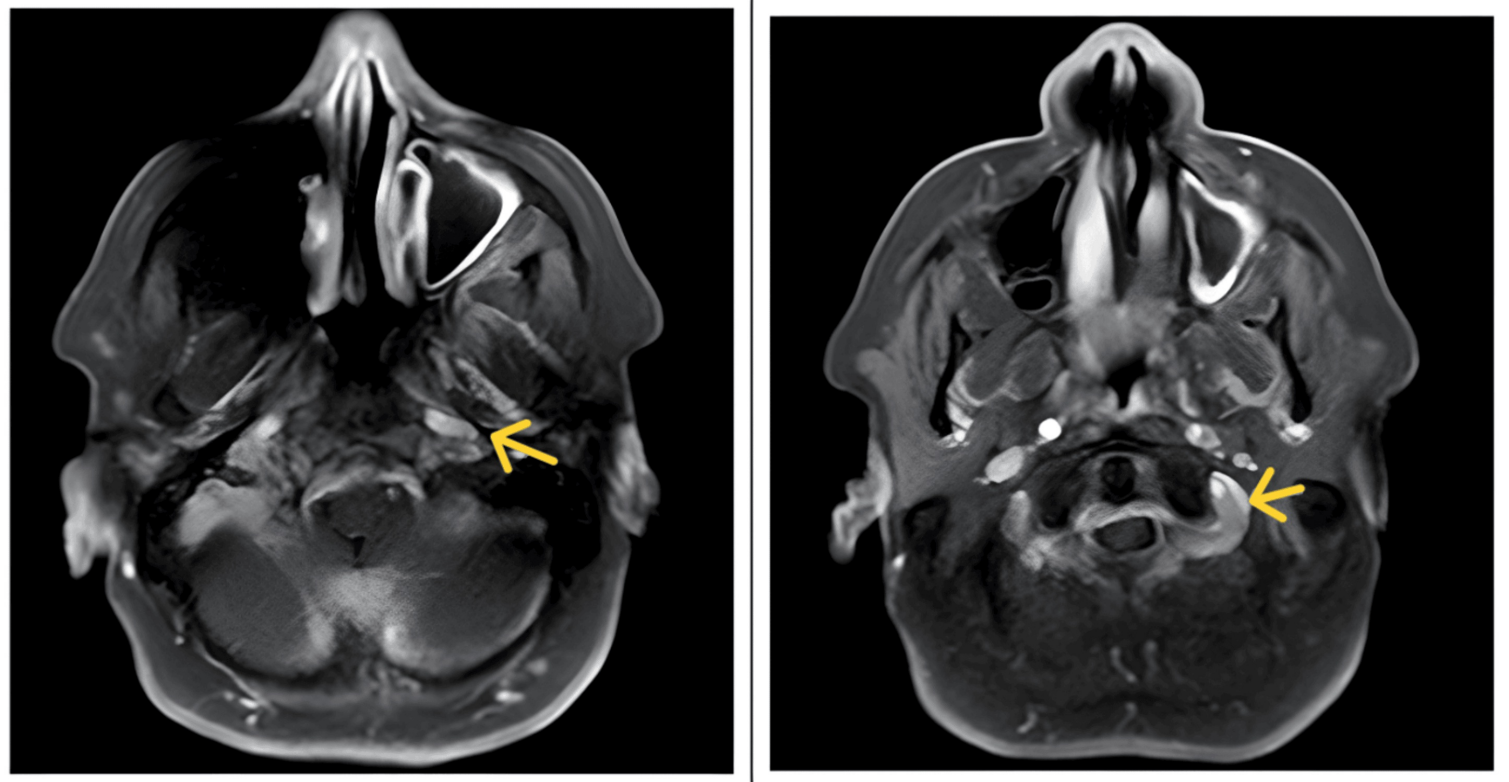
MRI with contrast axial view. Mild thickening and enhancement of bilateral IXth, Xth, and XIIth cranial nerves at the level of the medulla to the jugular foramen and XIIth nerve at the level of the medulla.
